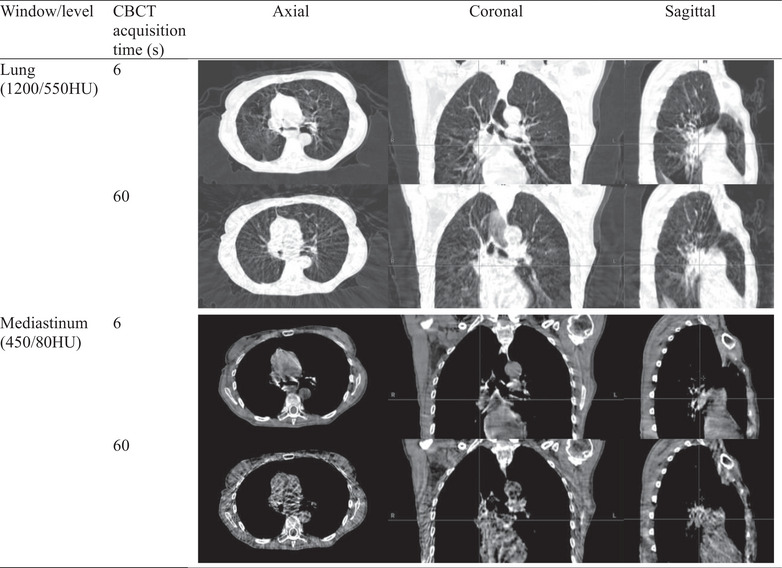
Motion artifact in 60‐second and 6‐second CBCTs for the same lung patient.
